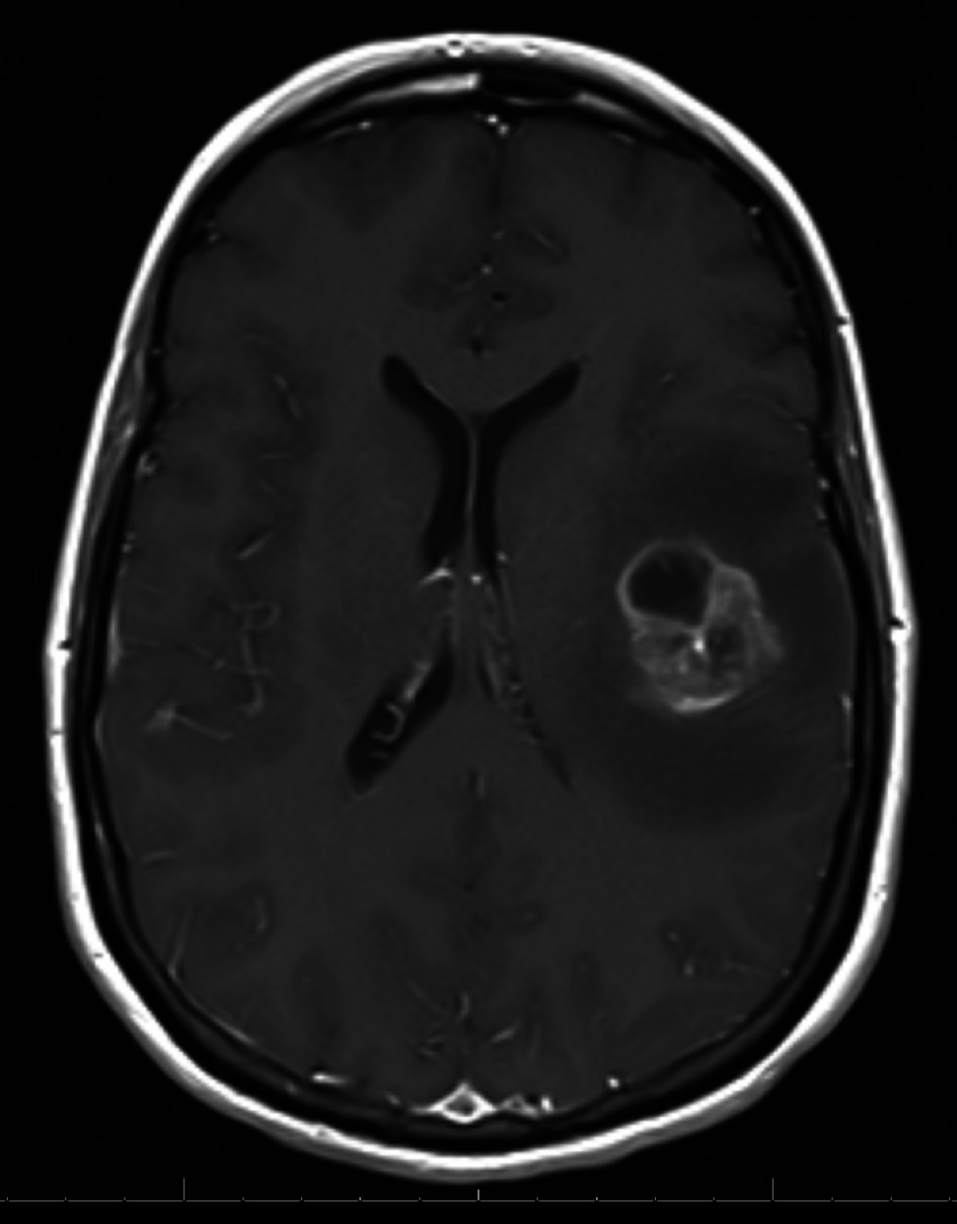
Magnetic resonance imaging (MRI) showing solitary metastasis in the left frontal lobe
